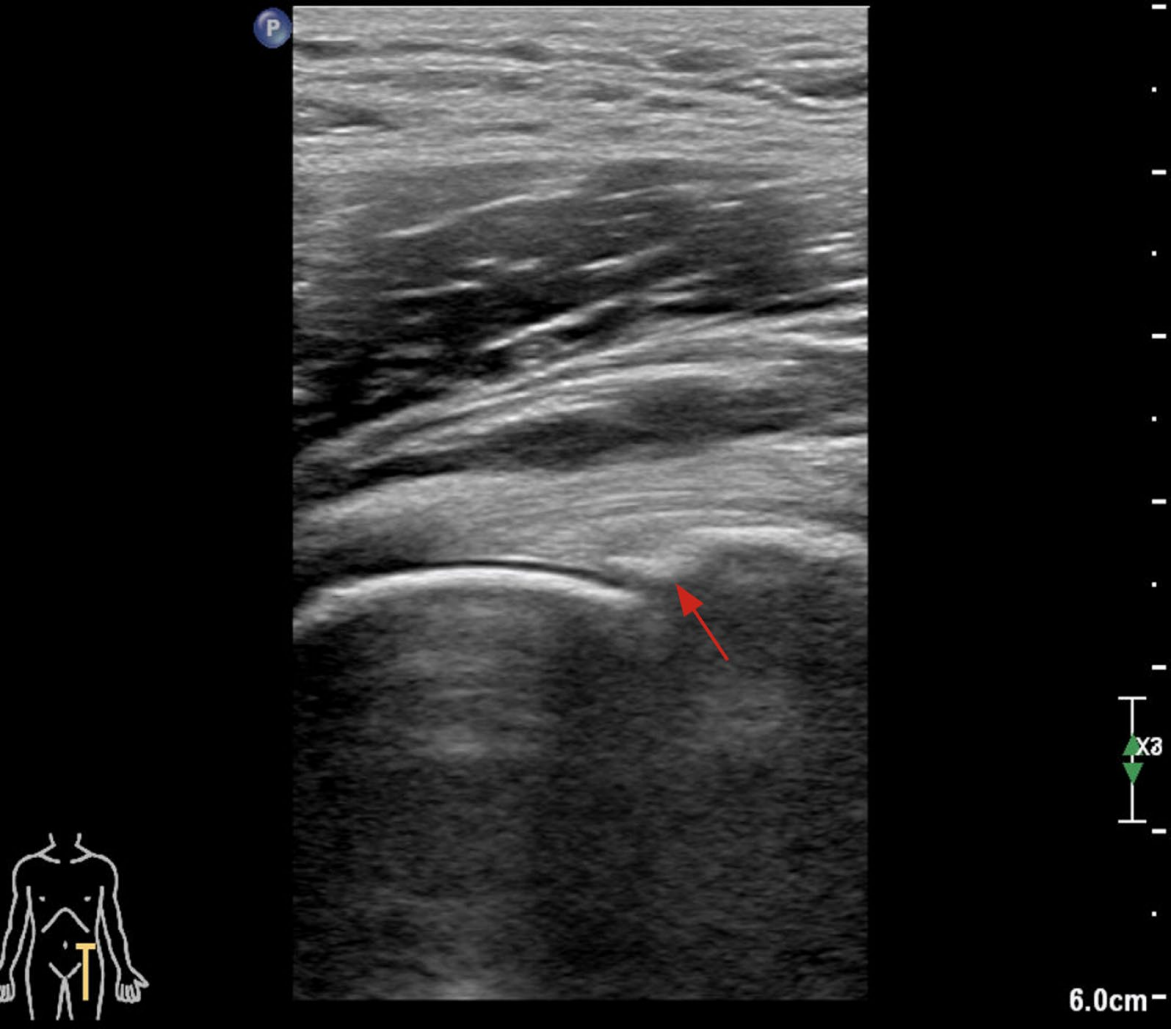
The ultrasonography imaging of labral lesion. The red arrow points to the labral edema
